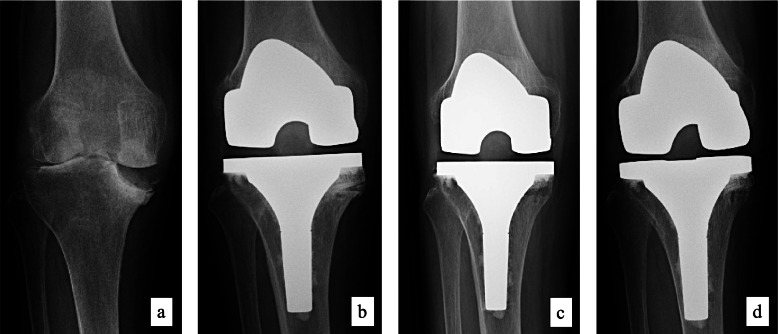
Pre- and postoperative follow-up radiographs of a 67-year-old woman patient (case 14) (a) Preoperatively. (b) Immediately after surgery. (c) Three months postoperatively. (d) Final follow-up
